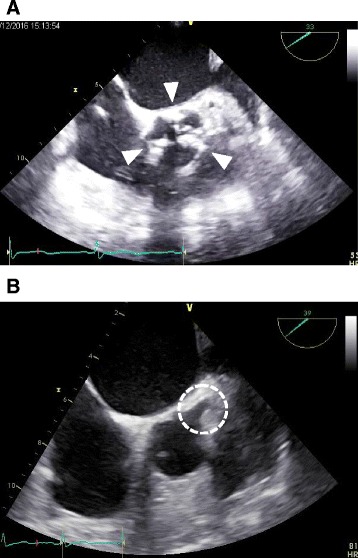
a Preoperative transesophageal echocardiography. Severe calcification was observed in all leaflets and aortic annulus (*arrowheads*). b Preoperative transesophageal echocardiography. The left coronary ostium was intact; there was no significant stenosis (*dotted circle*)
